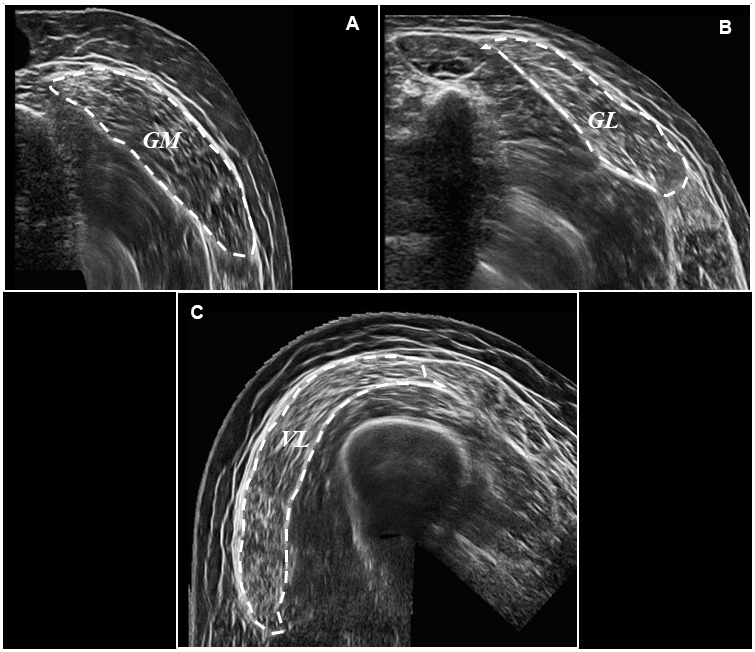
Representative ultrasound images following panoramic ultrasound imaging. Panel (A) represents a transverse image of *GM* CSA (outlined for effect) at 50% of muscle length, Panel (B) represents a transverse image of *GL* CSA (outlined for effect) at 50% of muscle length, and Panel (C) represents a transverse image of *VL* CSA (outlined for effect) also at 50% of muscle length.
